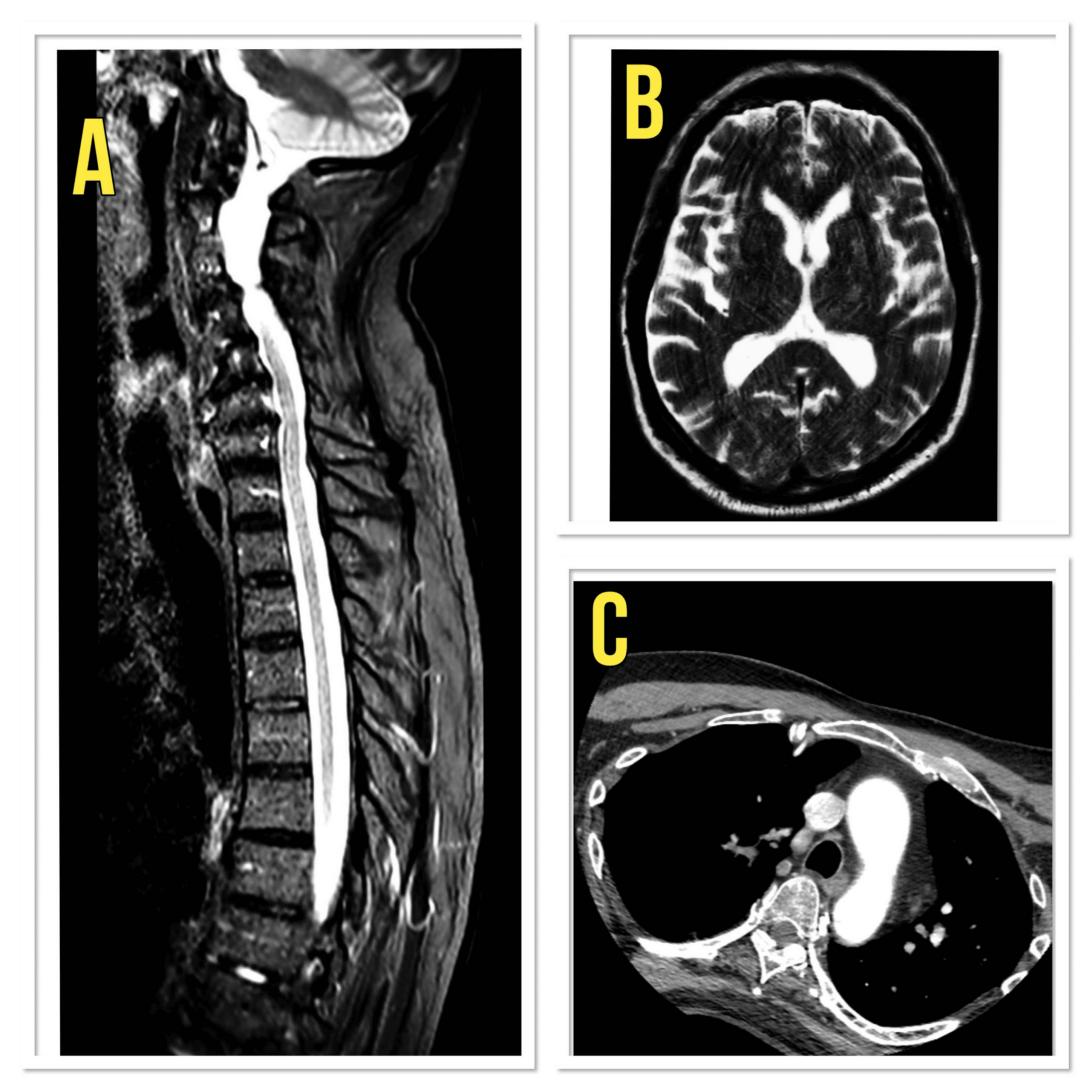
MRI whole spine, MRI head, and CT angiogram aorta of the patient (A) MRI whole spine, (B) MRI head, and (C) CT angiogram aorta. All investigations were reported as normal, and no pathology was identified in the imaging related to the symptoms of the patient.
